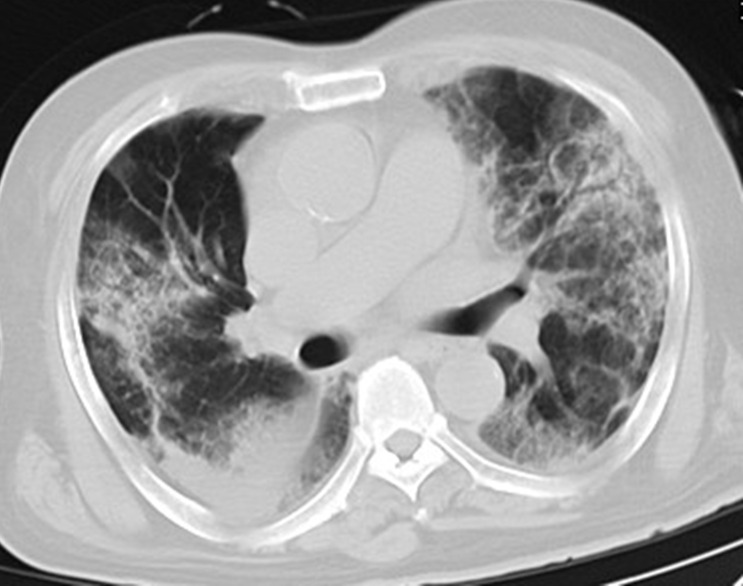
Initial chest CT of a 76-year-old man who presented with fever, cough, and dyspnea for 4 days. Bilateral extensive irregular patchy ground glass opacities (GGOs), strip opacities, consolidation, some with air bronchogram, with both central and peripheral distributions were observed. Mechanical ventilation treatments were used at admission, and the patient died of respiratory failure after 10 days of hospitalization.
